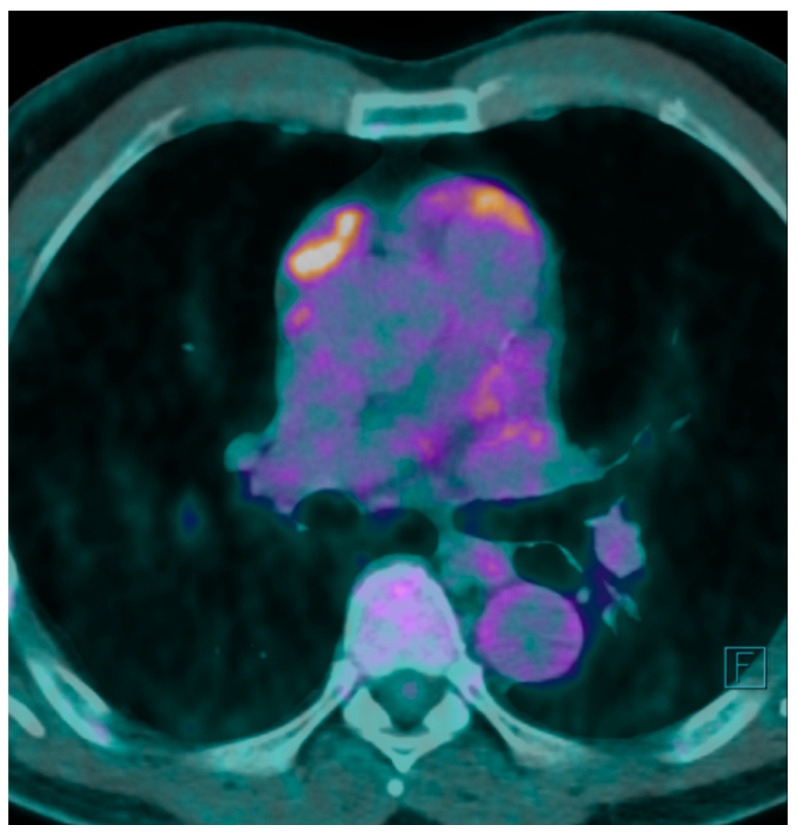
PET-CT shows uptake of F^18^ FDG in correspondence with anterior mediastinal mass. Brighter colors such as white, yellow and orange denote a higher F^18^ FDG uptake.
